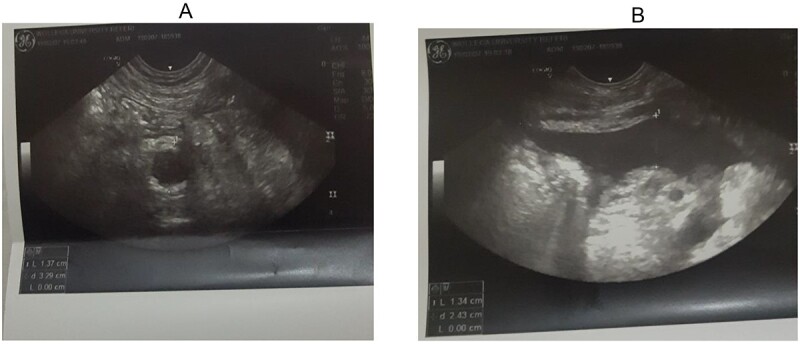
(A, B) Right lower quadrant ultrasound study showing dilated appendix and right lower quadrant fluid collection.
